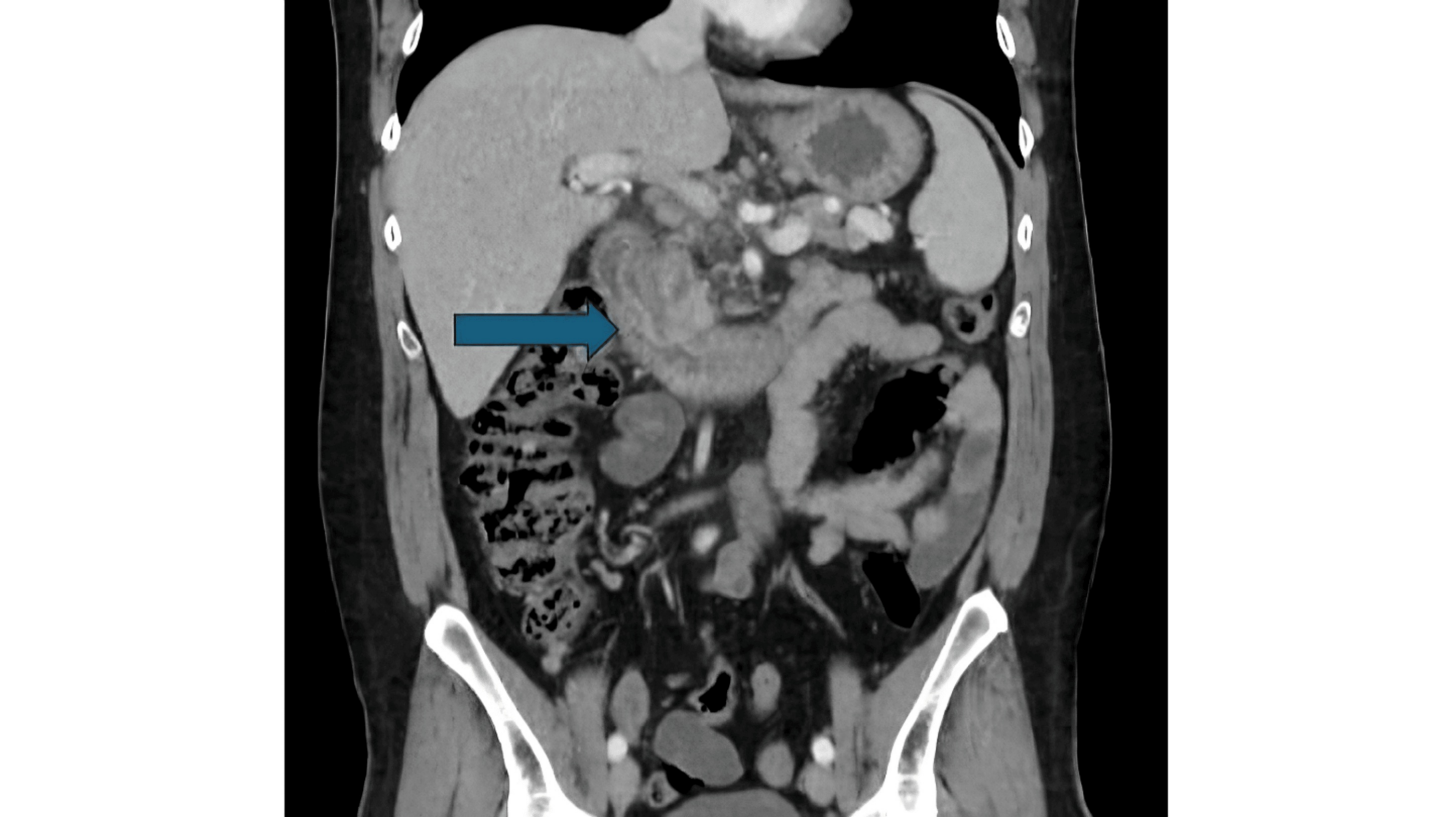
Computed tomography of the abdomen showing pancreatic ductal dilation prompting an MRCP. MRCP: magnetic resonance cholangiopancreatography.
